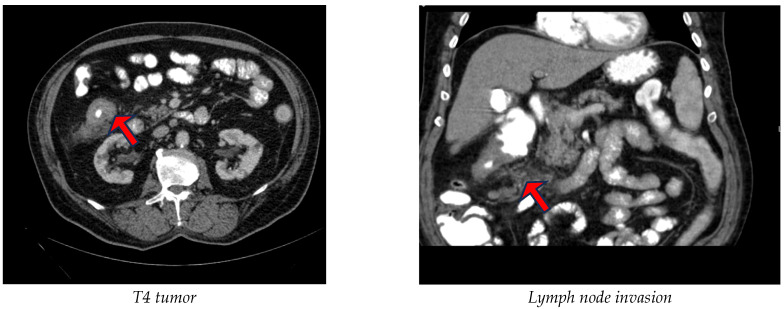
Abdominal CT scan showing T4 tumor and lymph node invasion. Arrows show T4 tumor and Lymph node invasion.
